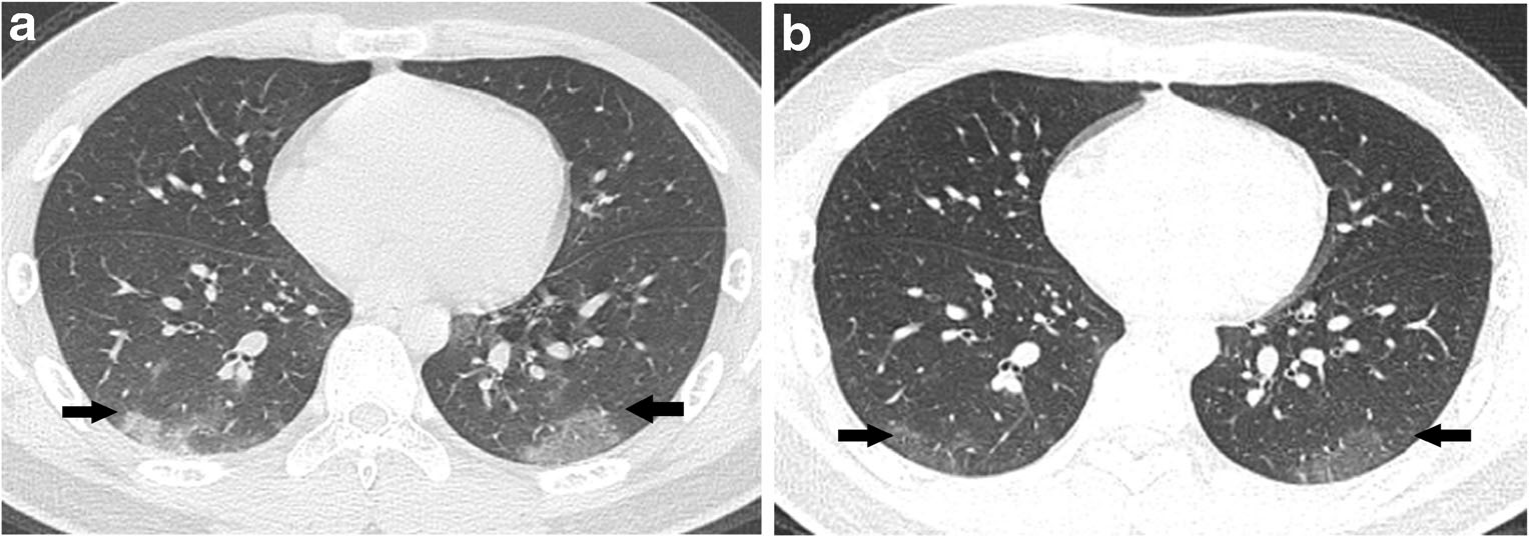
A 13-year-old symptomatic boy with COVID-19 with fever, cough and a history of exposure. a Axial thin-section non-contrast CT shows patchy peripheral ground-glass opacities in both lower lobes (*arrows*). b A follow-up CT image shows light ground-glass opacities after 5 days (*arrows*)
